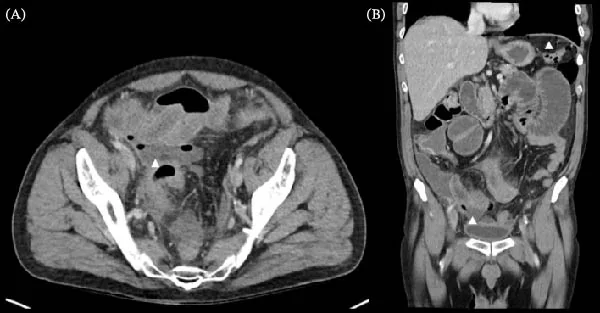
Repeat computed tomography scan of the abdomen and pelvis in the portal‐venous phase performed on day 3 of admission demonstrating coronal (A) and axial (B) views of possible small bowel obstruction with free gas and increasing pelvic collection (white arrows).
